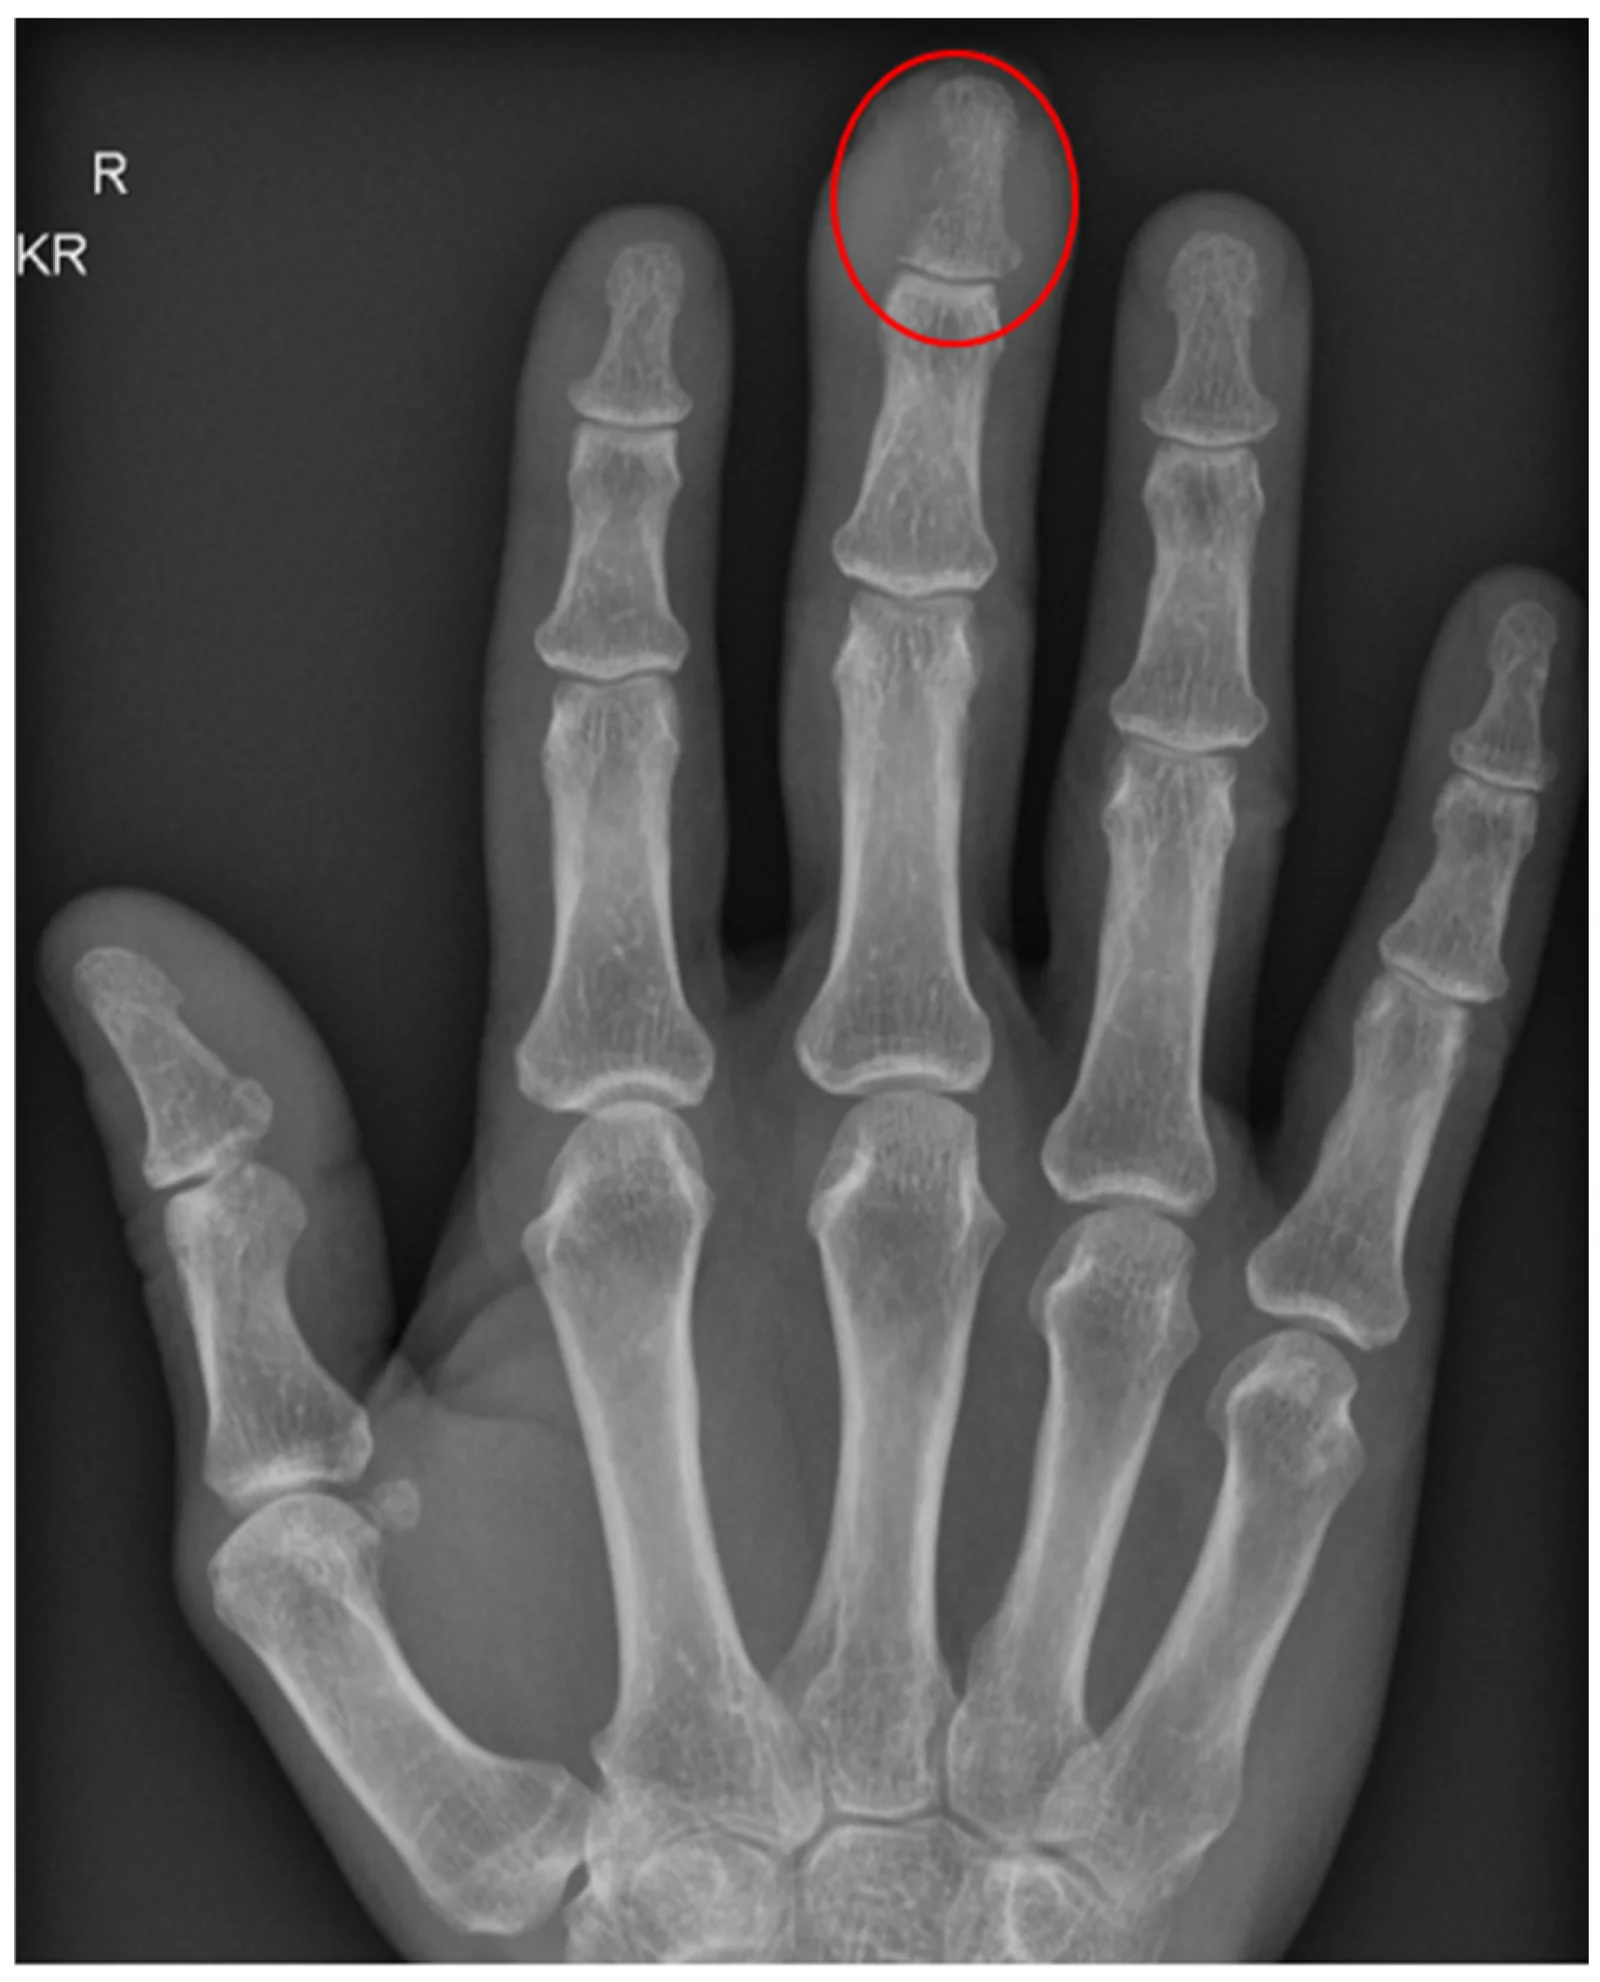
Anteroposterior radiograph of the right hand. The circled area demonstrates osteolytic bone destruction of the distal phalanx of the middle finger (digit III), consistent with distal phalangeal osteomyelitis.
